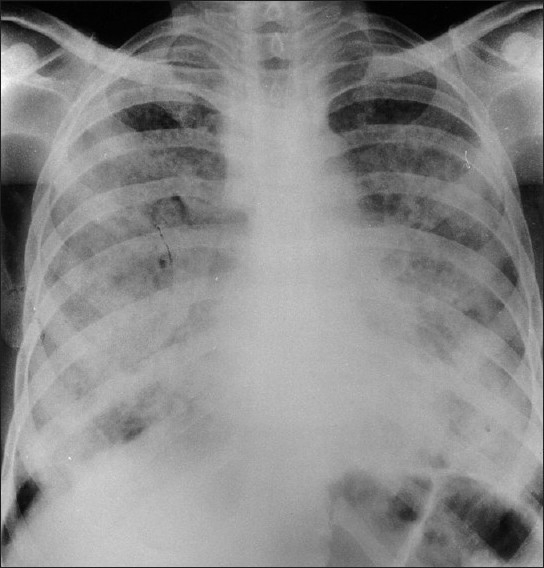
Postero-anterior view of chest radiograph showing nonspecific extensive bilateral patches of lung opacities, more marked in the lower zones
